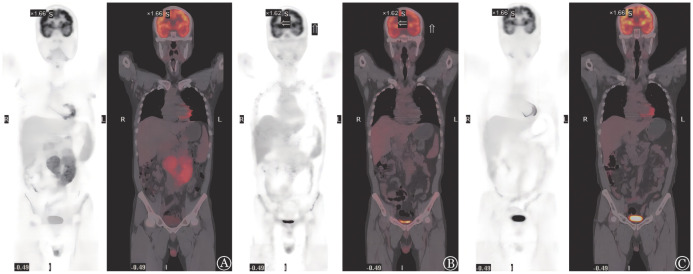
例2治疗前后PET-CT结果比较 A ZR-CHOP方案治疗前；B CAR-T细胞治疗后1个月；C CAR-T细胞治疗后3个月
